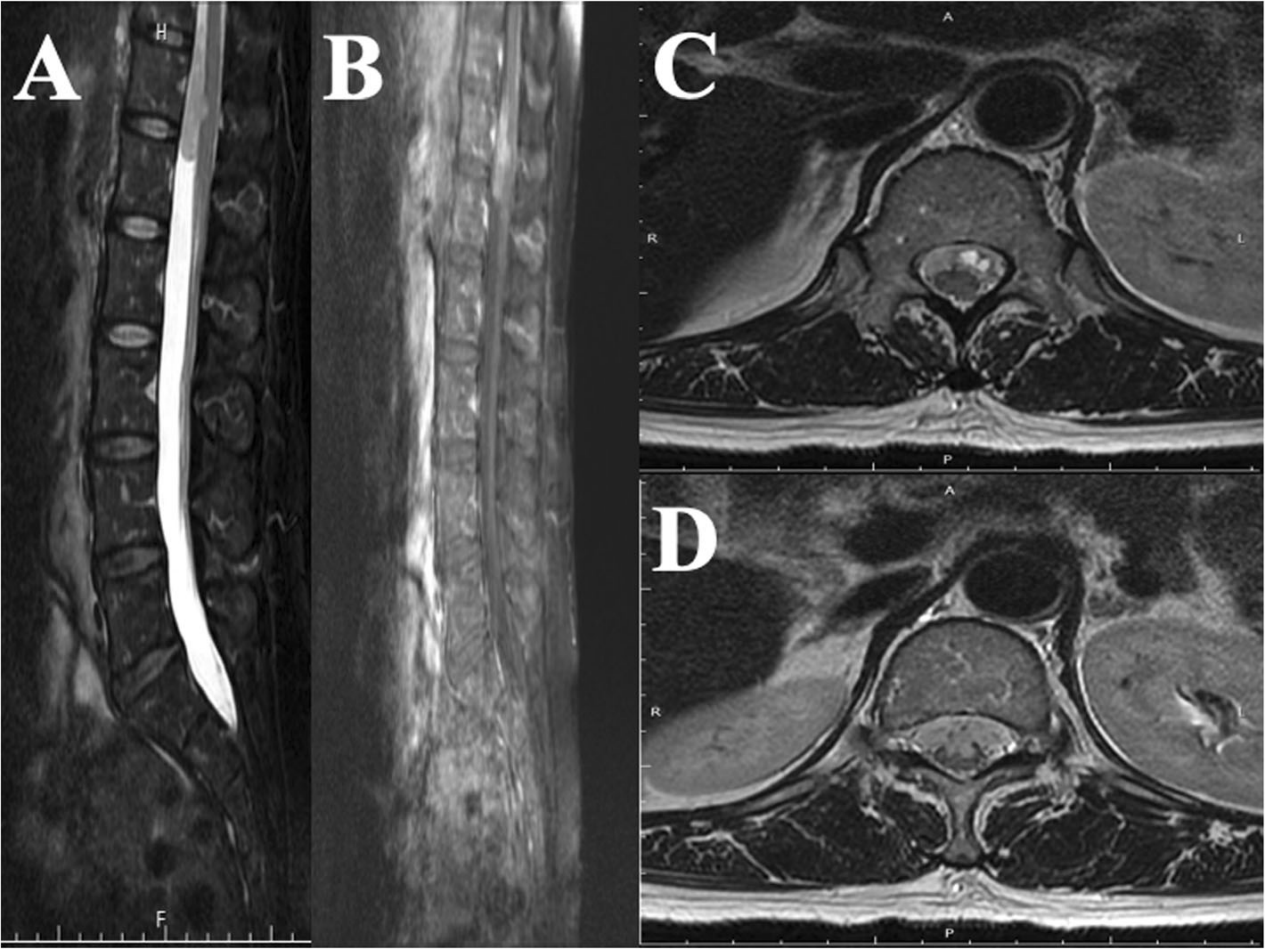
Spinal MRI results. a Sagittal T2-weighted image revealing a hypointense nodule at the T11–L1 level. b Postcontrast T1-weighted images depicting enhanced spinal tumor. c Axial section at the T12 level. d Axial section at the L1 level at the ventral aspect with conus medullary invasion
